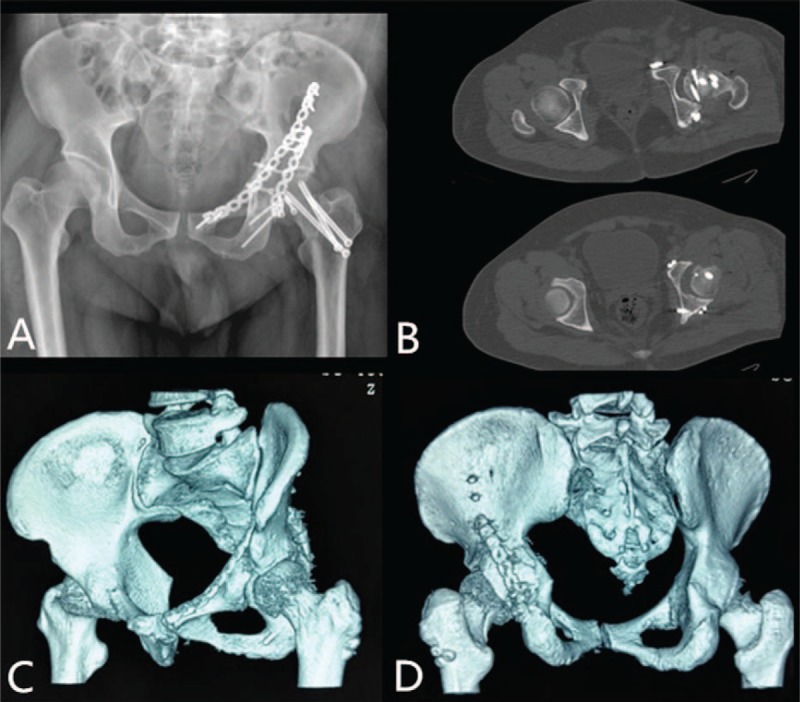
(A) Postoperative pelvic radiograph showing reduction of fractures. (B) Axial computed tomography showing no displacement of the femoral head or posterior wall. (C and D) Three-dimensional computed tomography showing good reductions of femoral head, femoral neck, and acetabular.
